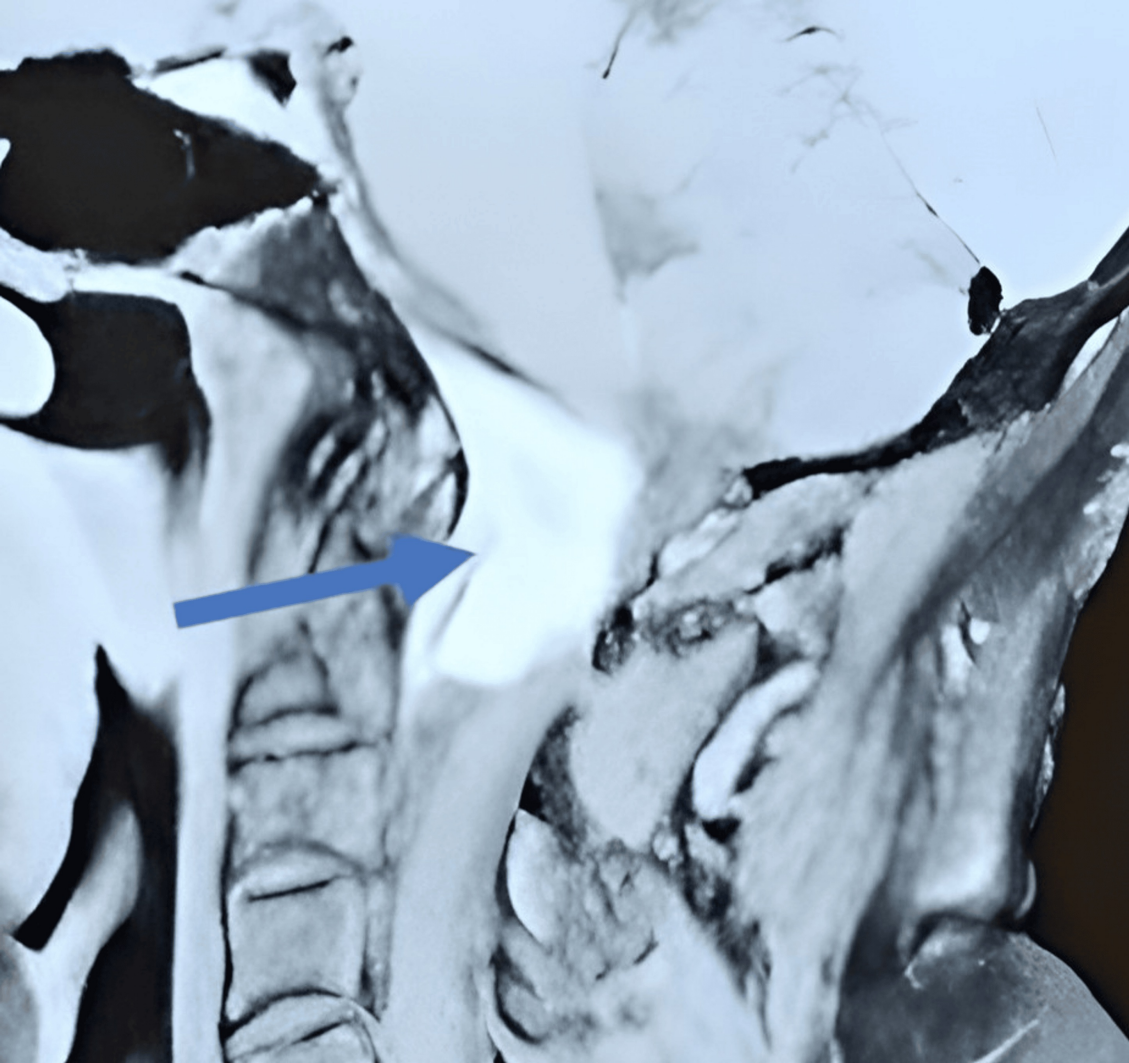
Sagittal T1-weighted MRI showing an extradural mass at the C1–C2 level (blue arrow).
